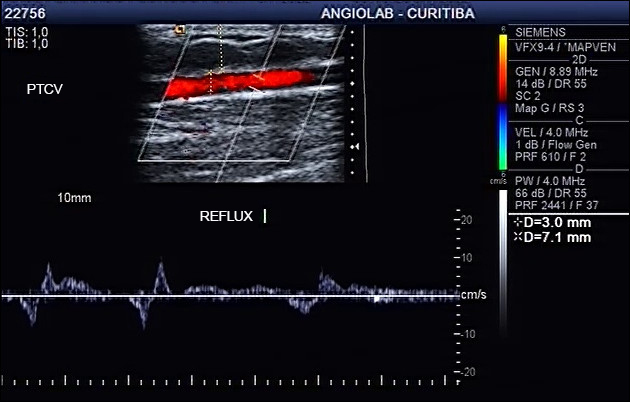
Ultrasound image of the Giacomini vein with reflux, with caliber of 3 mm and depth of 7.1 mm. PTCV = Posterior thigh circumflex vein (Giacomini Vein).
